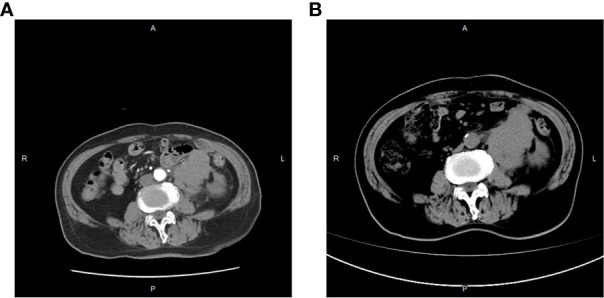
CT results. (A) CT result on April 25, 2022; (B) CT result on May 17, 2022.
